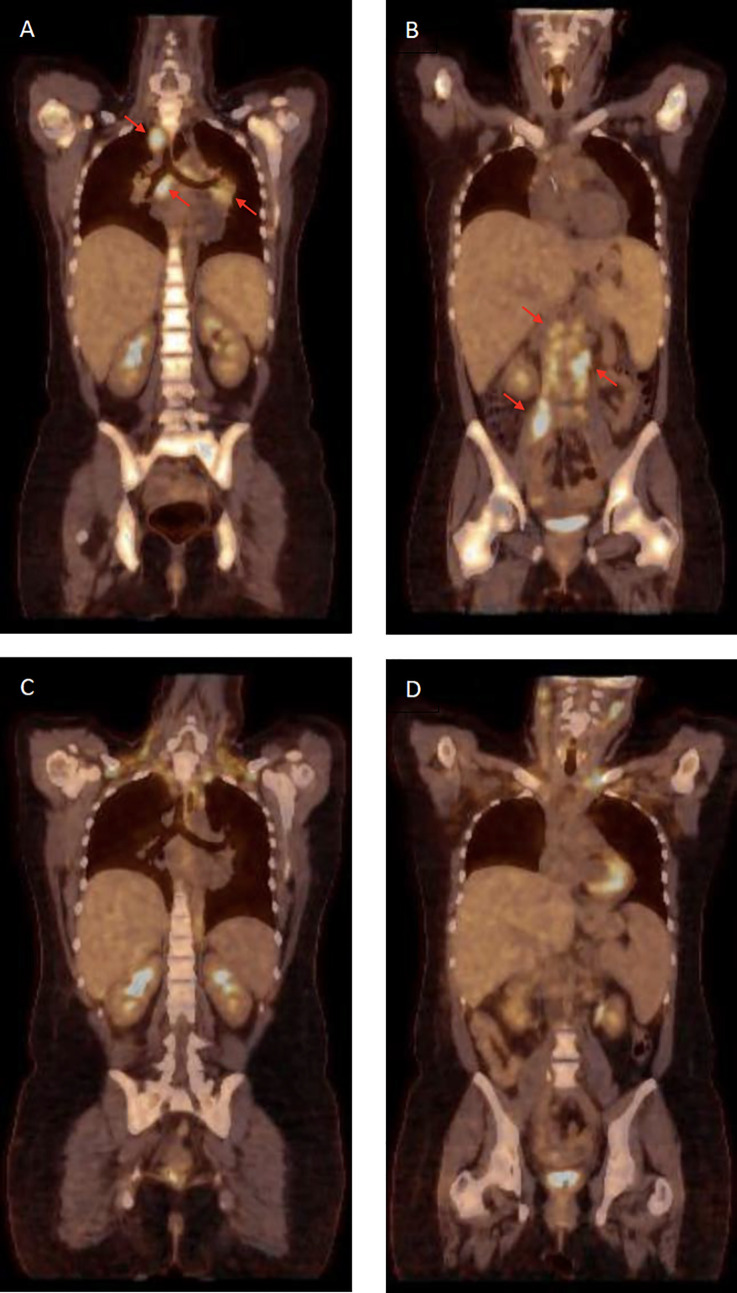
Positron emission tomography–computed tomography (PET-CT). (A, B) Disseminated lymphadenopathy due to non-tuberculous mycobacterial (NTM) infection (red arrows) and bone-marrow activation and hepatosplenomegaly due to hemophagocytic lymphohistiocytosis (HLH) at diagnosis. (C, D) Control PET-CT following allogeneic hematopoietic transplantation (day +240) and after 12 months of tuberculostatic therapy demonstrating complete remission of NTM-related lymphadenopathy and remission of HLH-associated hematopoietic activation.
